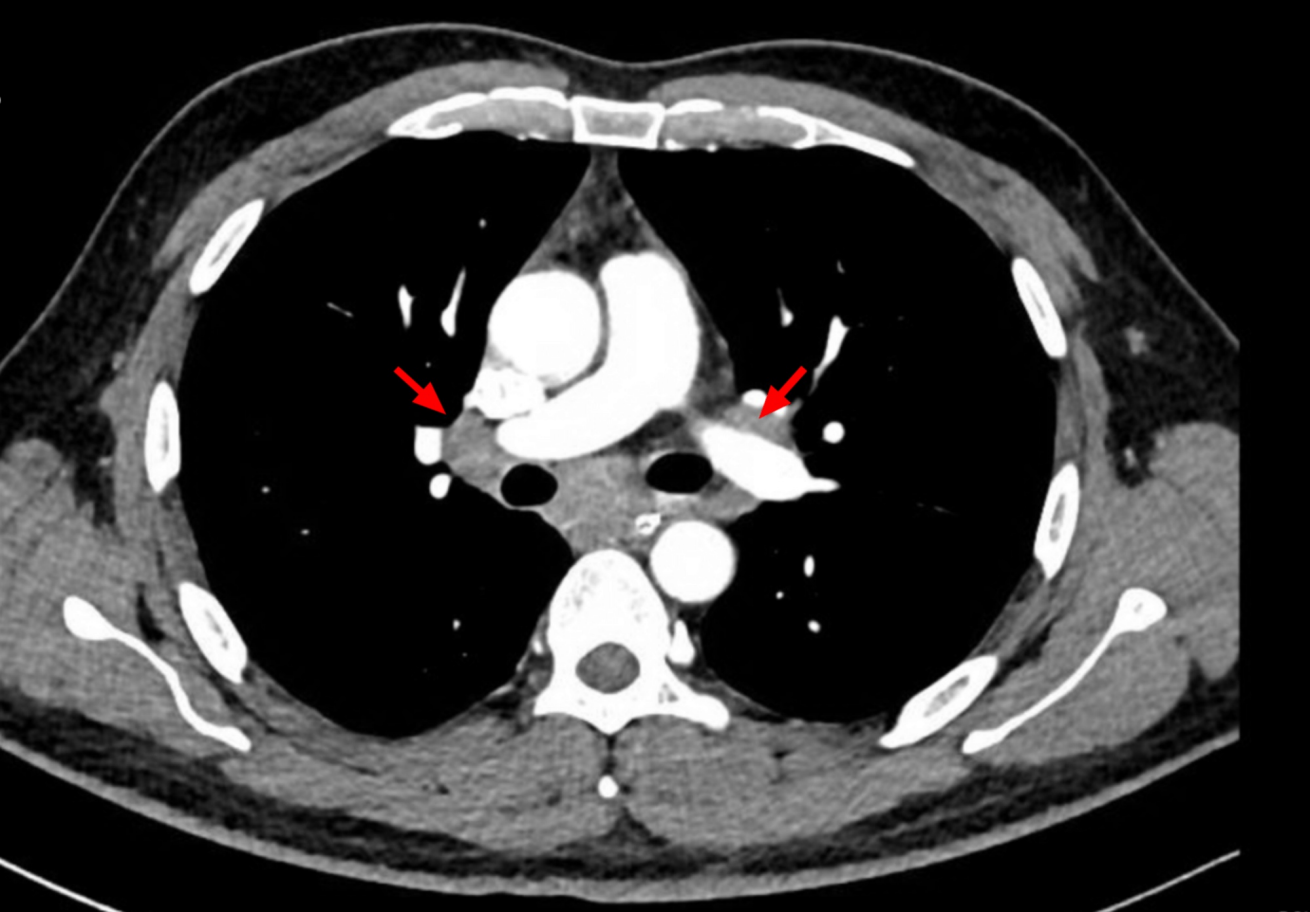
Computed tomography (CT) scan of the thorax Red arrows: paratracheal and hilar lymph nodes.
